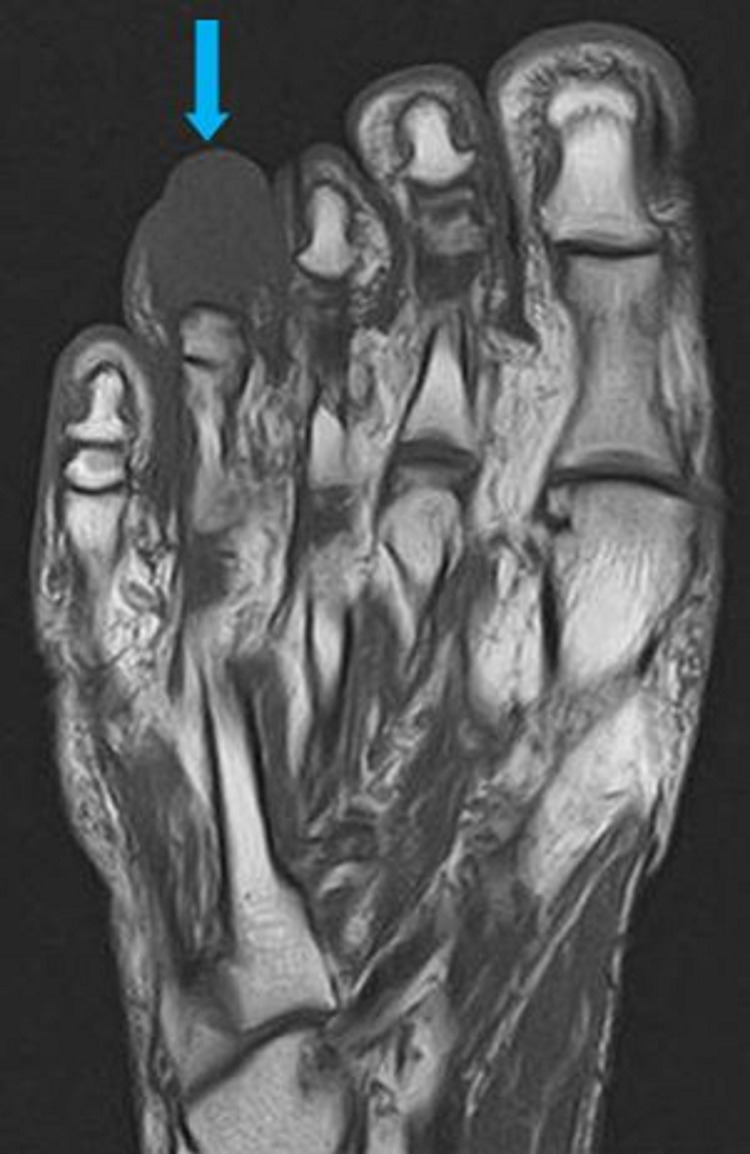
Coronal view on the MRI T1 sequence visualizing the mass on the distal phalange of the fourth toe (blue arrow)
